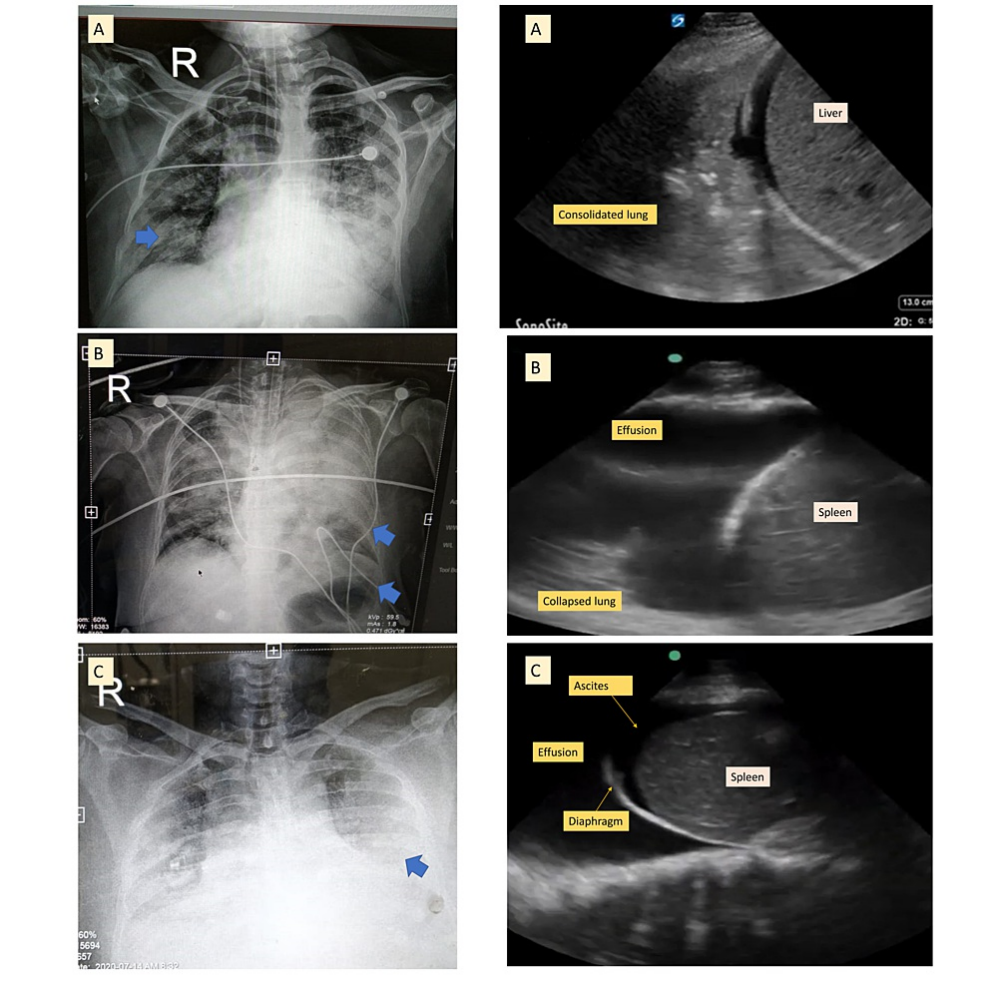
Visualization of different pneumonia patterns on chest radiograph in patients with cirrhosis and correct interpretation using lung ultrasound
